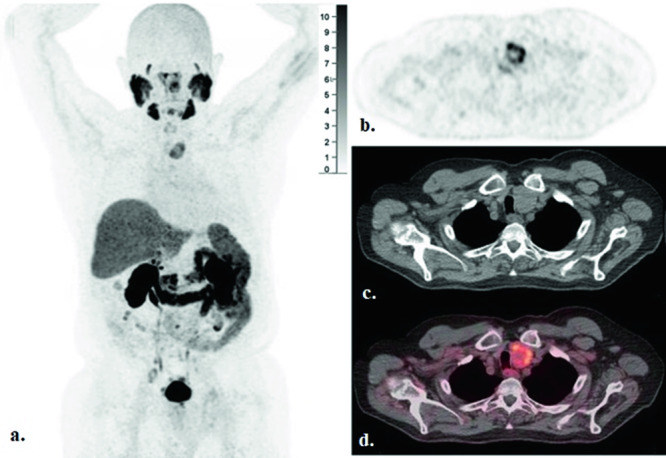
F-18 DCFPyL PET/CT imaging obtained before pembrolizumab start due to disease progression, with concurrent prostate-specific antigen level of 281 ng/ml in Spring 2023. a MIP, b Axial PET, c Axial CT, d Axial fusion PET/CT with PSMA uptake in a left thyroid nodule with dimensions of 3.6 × 3.0 cm (SUVmax: 8.2).
